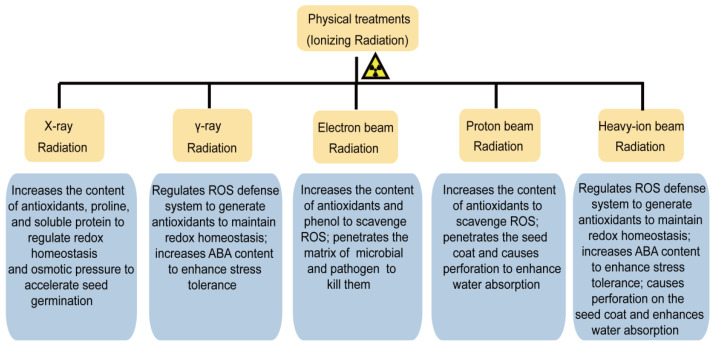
X-ray and γ-ray, electron beams, proton ions, and heavy-ion beams of physical agents used for seed priming and their mode of action.
